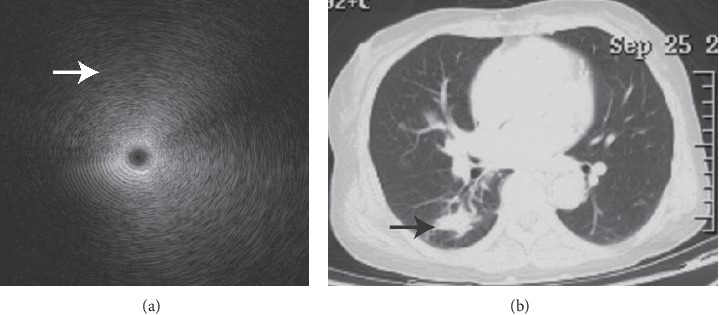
Homogenous internal echoes. Image of a 72-year-old woman with a peripheral consolidation lesion over the right lower lung lobe by chest radiography ((b) black arrow) with parrot chlamydia pneumonia identified by mNGS. EBUS demonstrated homogeneous internal echoes without margins (a). The particles displayed a formation of concentric circles around the echo probe, and entire images exhibited a sense of gradation. The particles lengthened to form a very short arc of the circumference, particularly in the outer part (white arrow).
